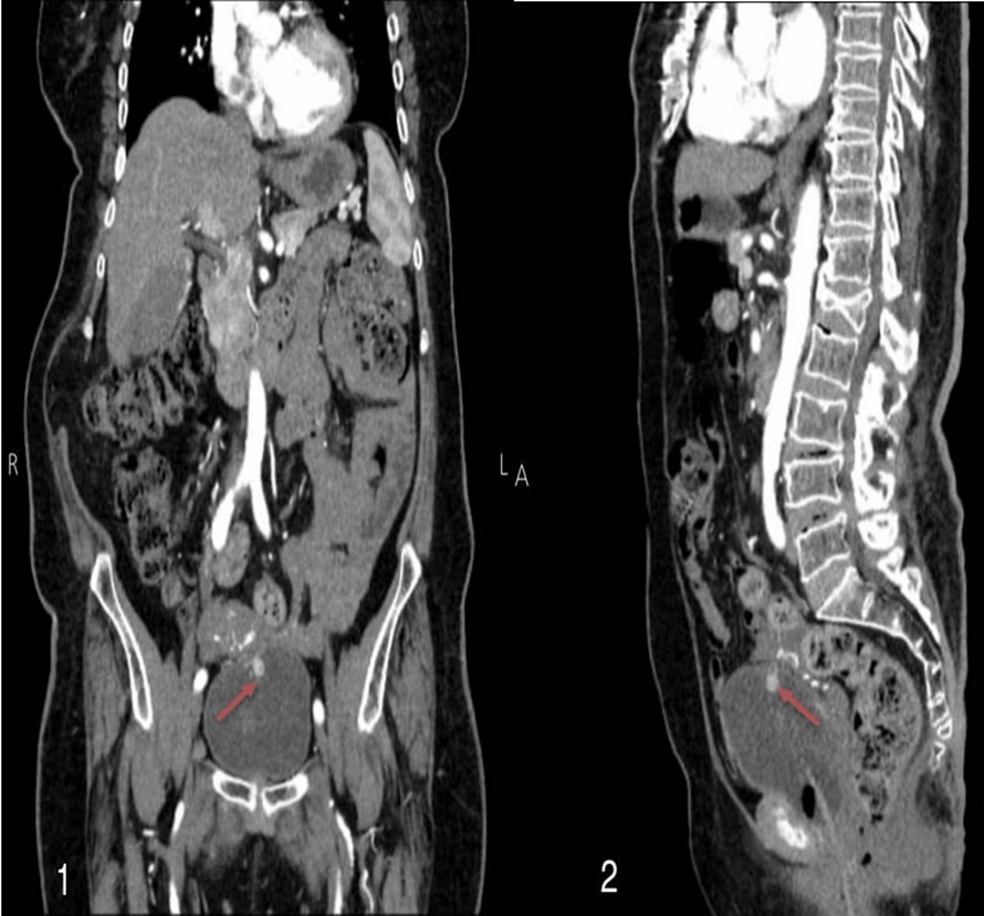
CT scan of the urinary tract showing a polypoid lesion protruding from the dome of the urinary bladder. CT scan of the urinary tract showing a vividly enhanced polypoid lesion measuring about 1 cm protruding from the dome of the urinary bladder. (1) Arterial phase - coronal section; (2) Delayed phase - sagittal section.
